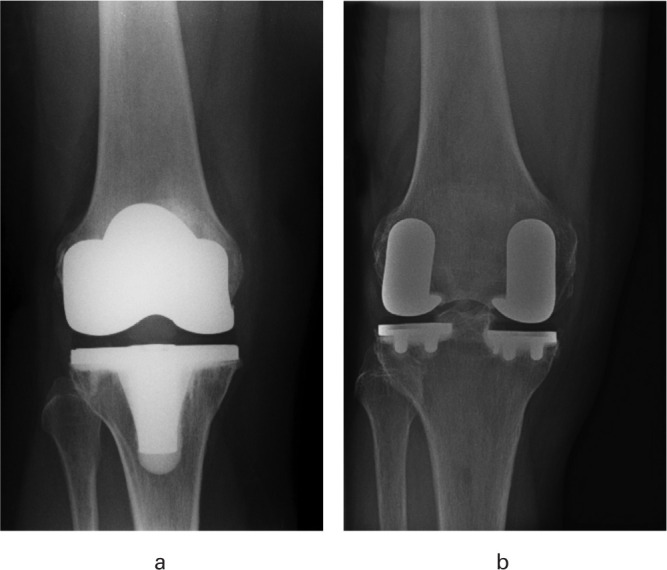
Anteroposterior radiographs of a) total knee arthroplasty using a NexGen LPS-Flex, and b) bi-unicompartmental knee arthroplasty using the medial and lateral Restoris compartmental implants.
